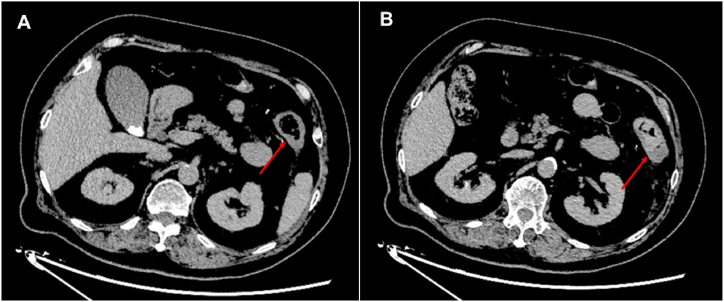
Transverse CT-enhanced images show diffuse bowel wall thickening in the splenic flexure of the colon (A) and bowel wall thickening in the descending colon (B).
